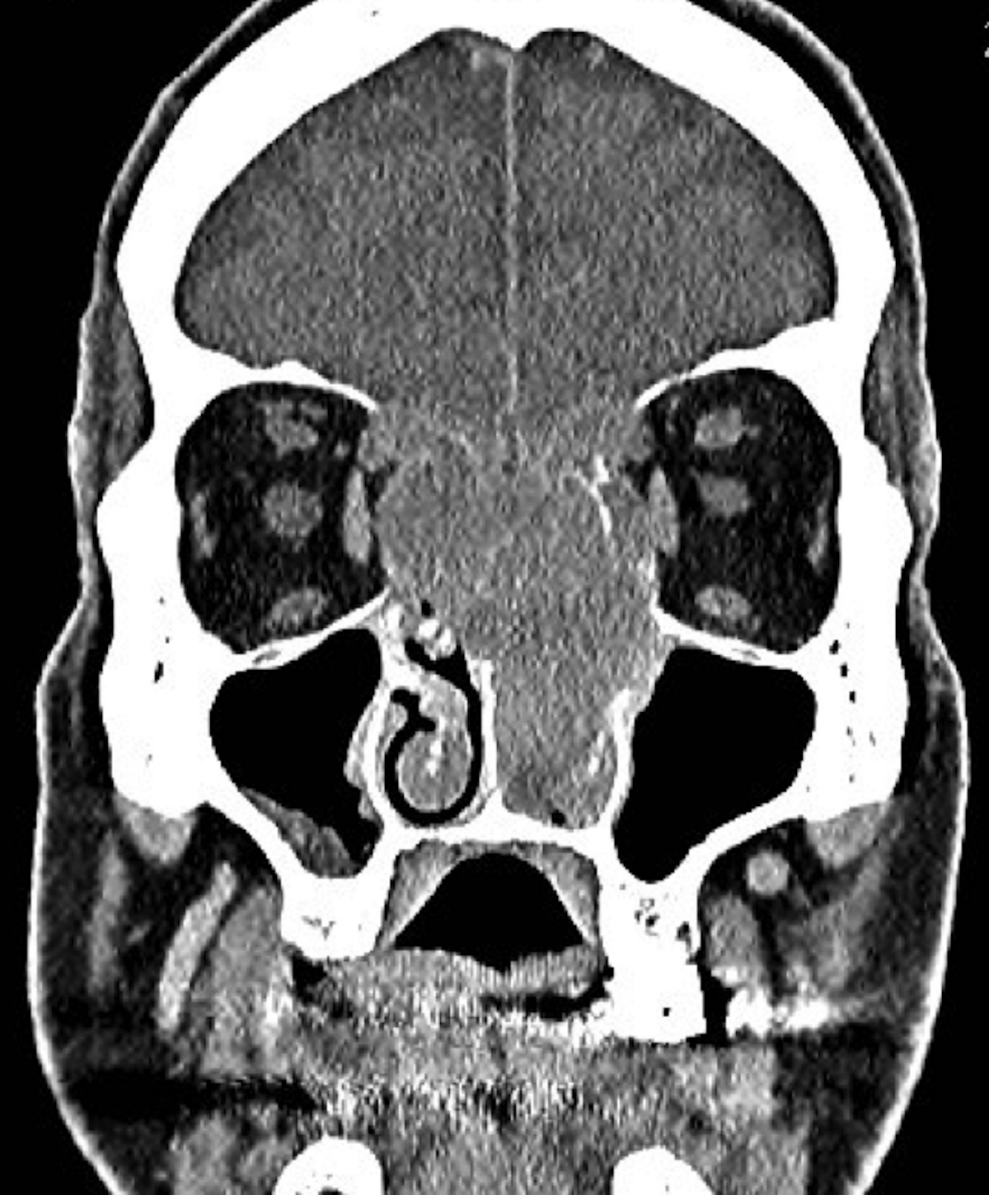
Contrast-enhanced computed tomography scan Coronal cut showing the lesion eroding the bilateral lamina papyracea and the cribriform plate and encroaching on the medial recti muscles.
